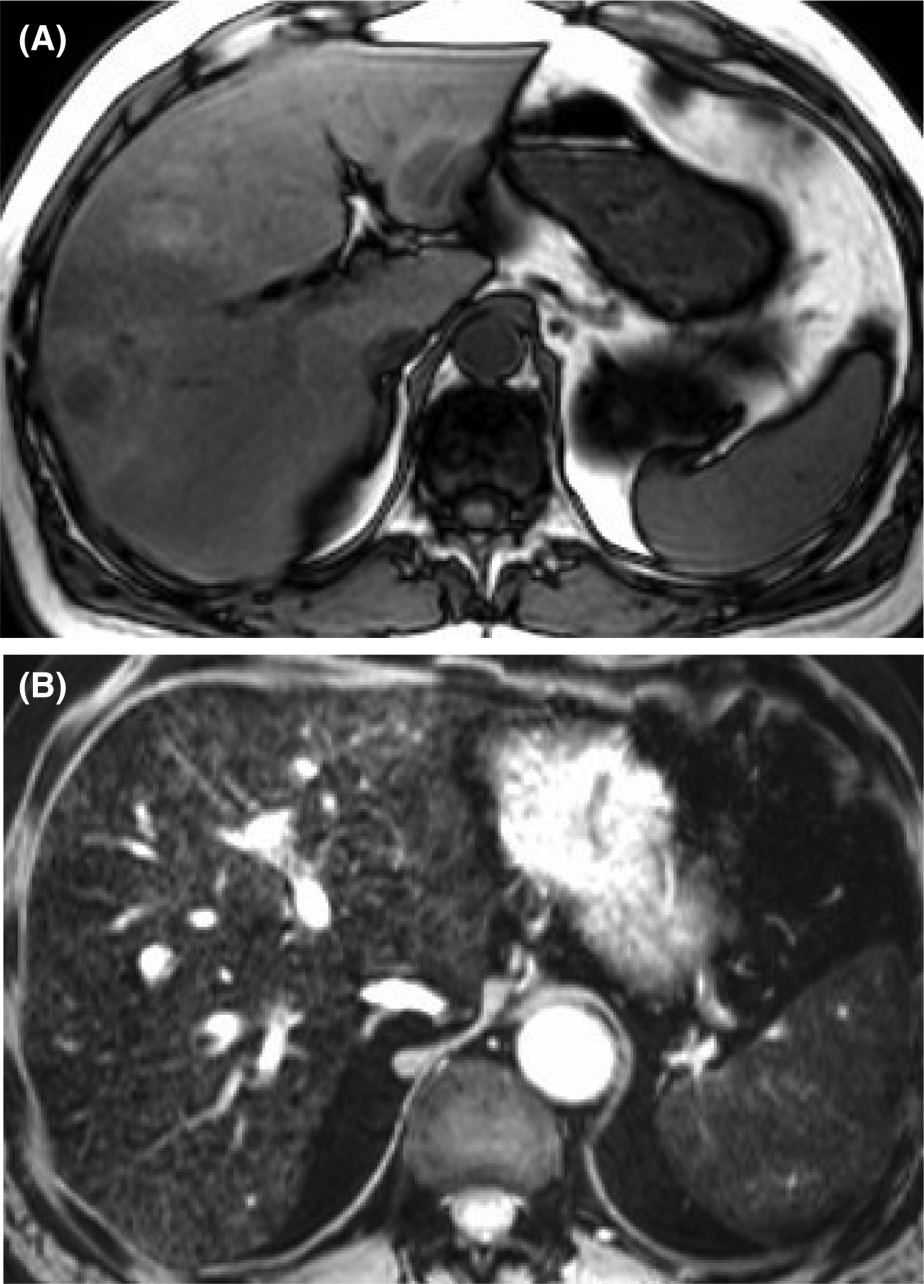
Liver MRI shows steatosis following irinotecan‐based chemotherapy (A) and sinusoidal obstructive syndrome (SOS) after oxaliplatin (B).
